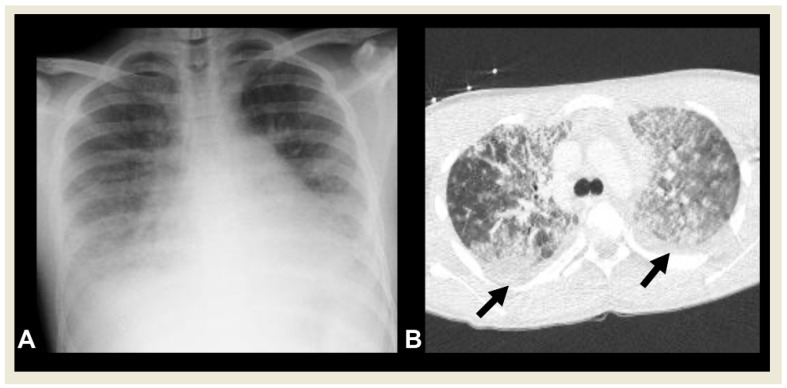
A 15-year-old boy with PARDS. (A) Chest radiography shows bilateral, diffuse lower lung opacities, and pleural effusions. (B) CECT shows bilateral ground-glass and alveolar opacities, and interlobular septal thickening with concomitant pleural effusions (arrows).
